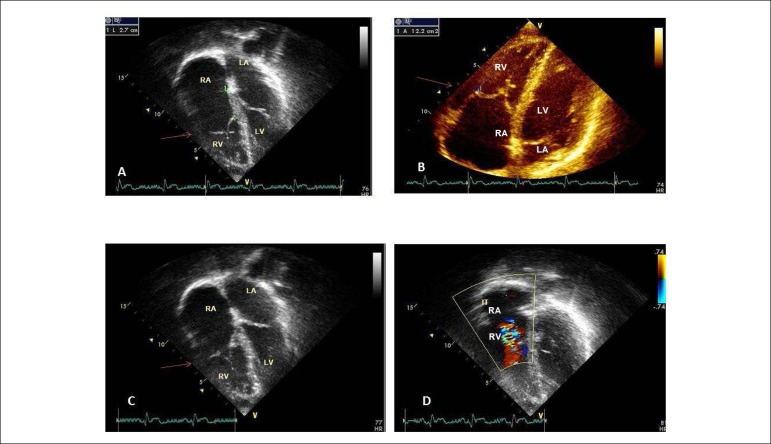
Echocardiogram shows, in the 4-chamber subcostal section, the septal
